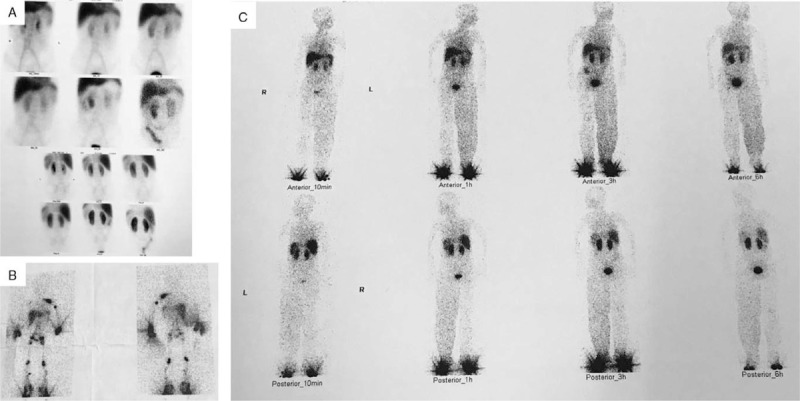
^99m^Tc-labeled dextran lymphoscintigraphy and ^99m^Tc-labeled human serum albumin scintigraphy. (A) Intestinal loss protein imaging documented protein loss into the intestinal lumen in the right lower quadrant of the abdomen. (B) Lymphoscintigraphy shows the absence of lymphatic drainage vessels in the right upper limb and lymph nodes in the right axillae. (C) Lymphoscintigraphy shows lymphedema in both legs, thickened left leg, the absence of lymphatic drainage in lower extremities, and lymph nodes in the popliteal fossa and above the bilateral inguinal ligaments.
